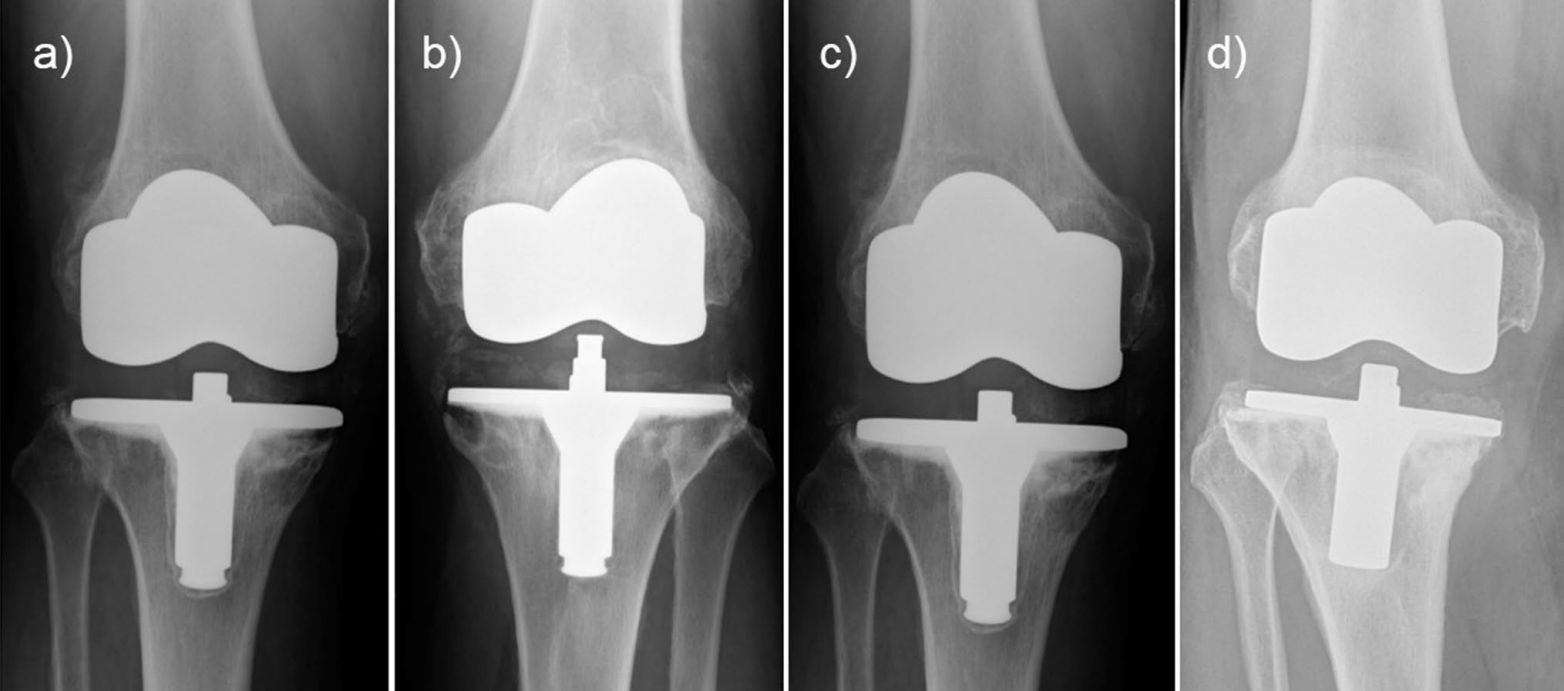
High-flex knees with aseptic loosening of the cemented tibial prosthesis after a 7 years (zone 1 and 5), b 8 years (zone 1), c 14 years (zone 1), and d 16 years (all zones with dislocation) using the Knee Society Roentgenographic Evaluation and Scoring System
